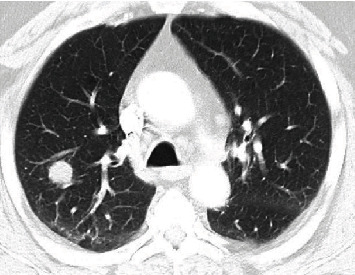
A large 19 mm nodule in the right upper lobe lung on noncontrast CT chest.
